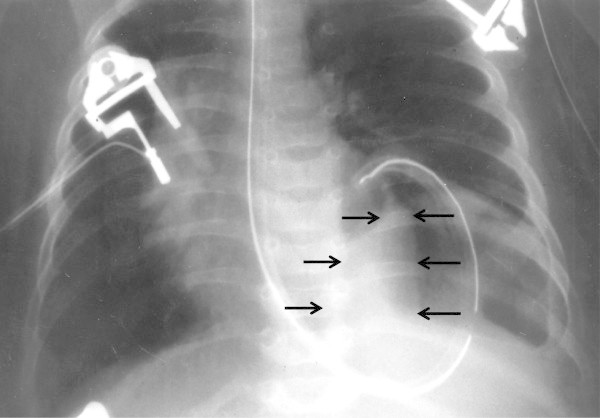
Frontal chest radiograph demonstrating nasogastric tube in the left hemithorax with displacement of the mediastinum to the right. Note the normal cardiac shadow. The left lobe of the liver is in the chest and is demarcated by black arrows.
